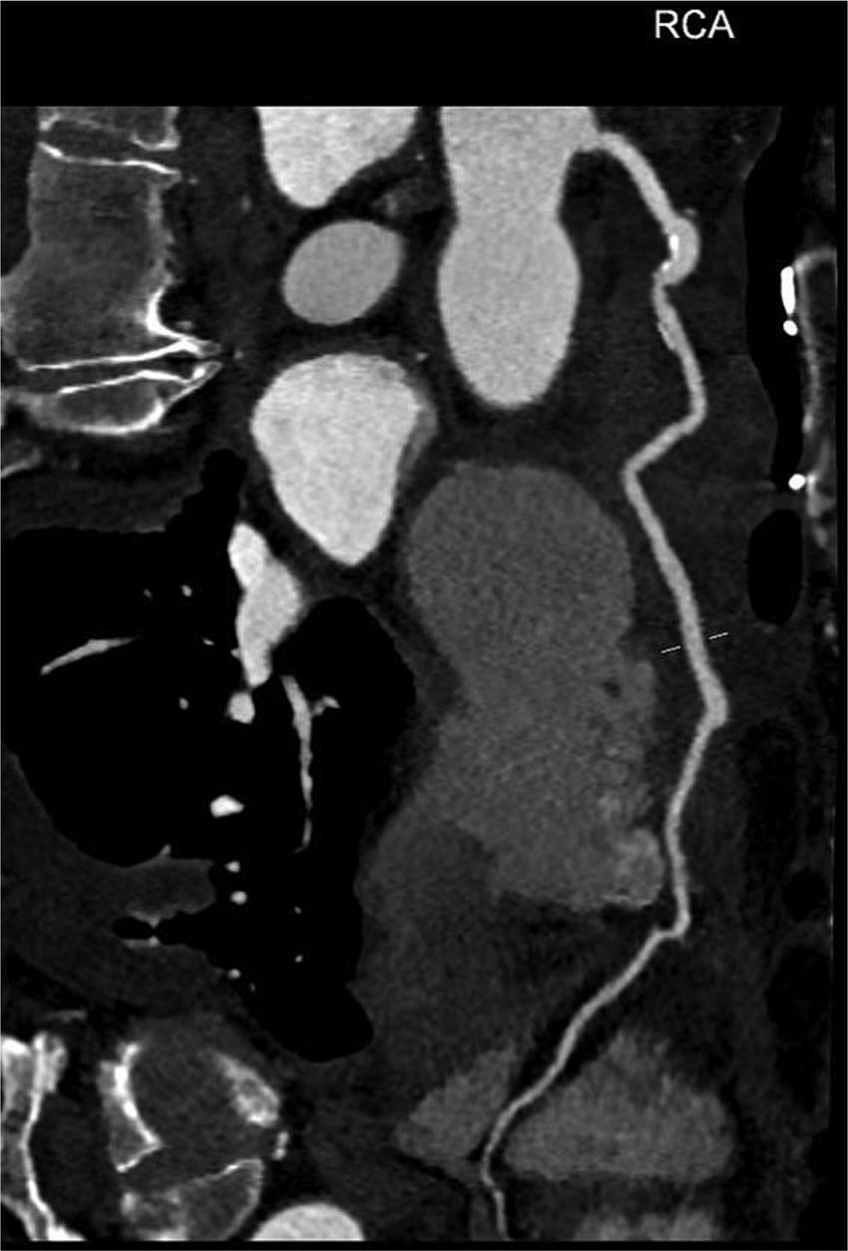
Reconstructed path of right coronary artery including vein graft via aneurysmal sac from post-operative CT coronary angiogram.
